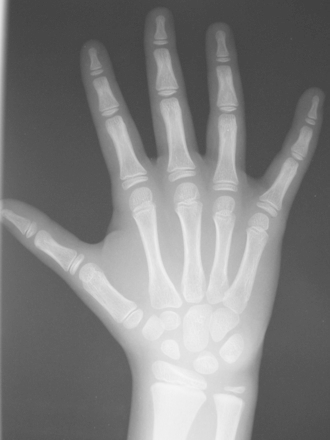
Mirrored left-hand/wrist radiograph.
